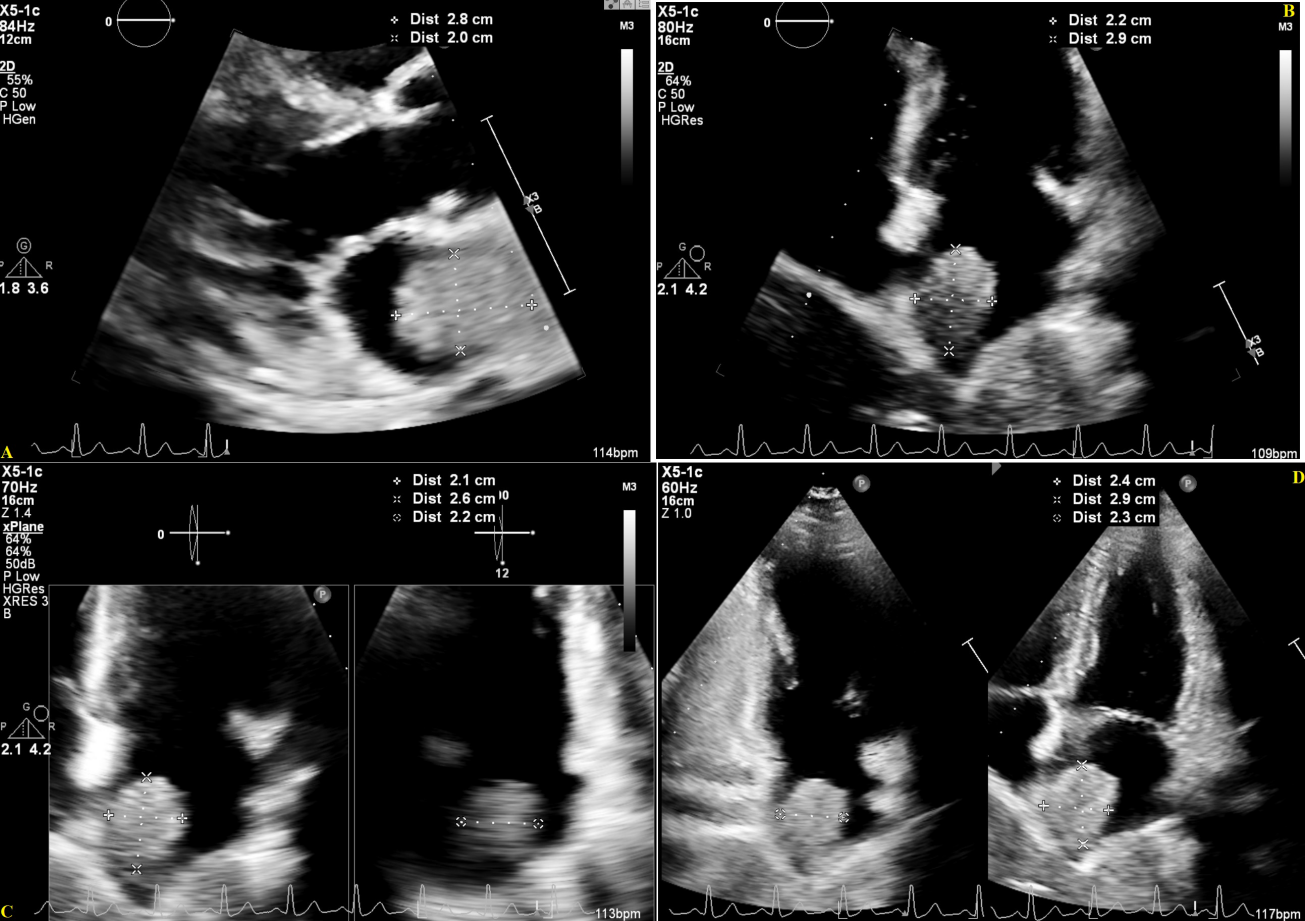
Transthoracic Echocardiogram showing a large echogenic mass in the Left Atrium, in the parasternal long-axis (A) and apical multi-chamber views (B–D), July 26, 2022, with measures estimating a mass size of 2.6-2.9 cm x 2.0-2.4 cm.
